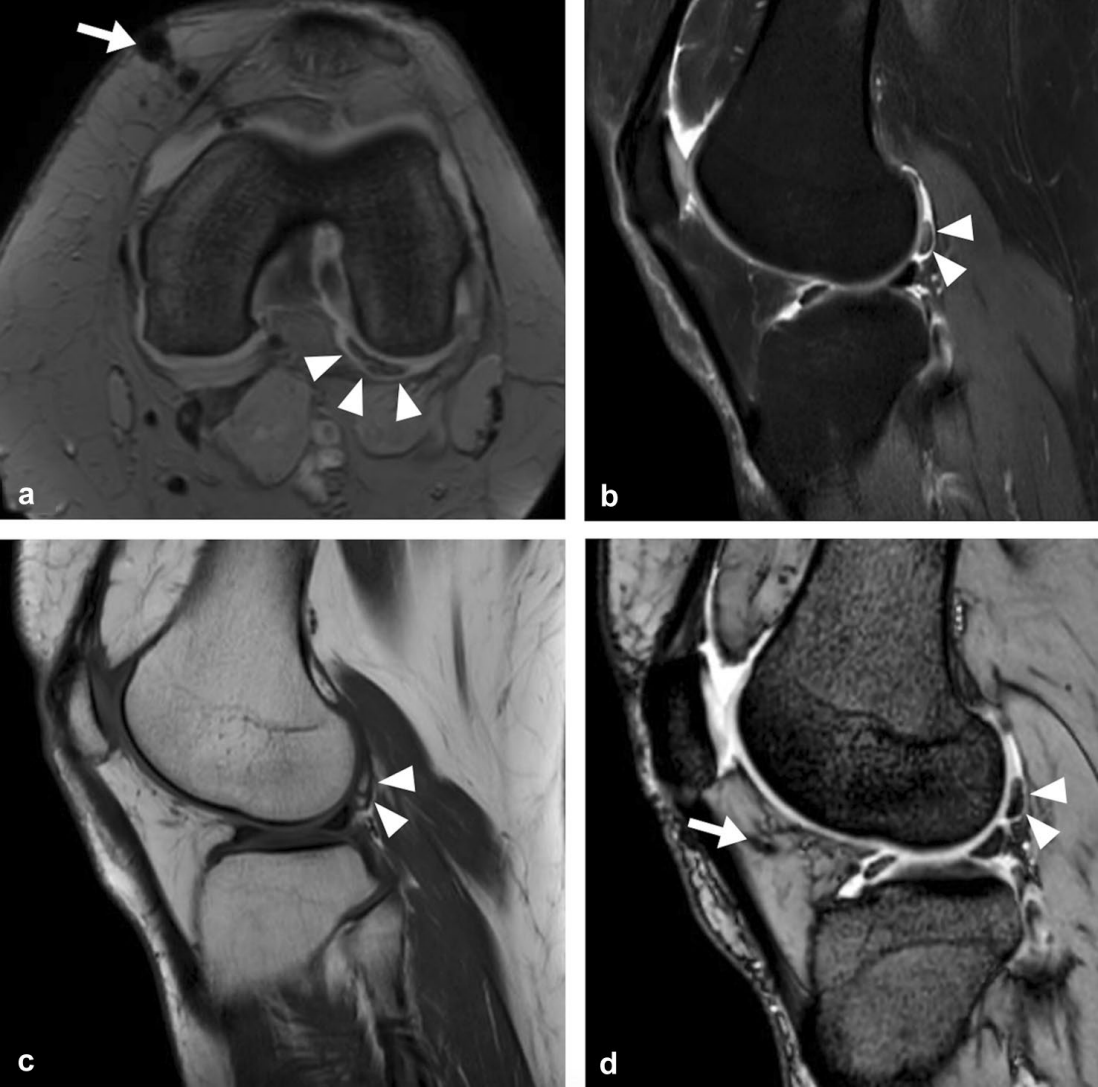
A T2-weighted sm-FFE axial image (Achieva 1.5 T Philips MRI scanner, with dStream technology). A large loose adipocyte intraarticular body in the posterolateral corner circumferentially surrounded by fluid effusion. The intraarticular body is hypointense, well defined (white arrowheads). On the medial anterior side the magnetic susceptibility artifact due to surgical scar (white arrow). B-D sagittal plane (Achieva 1.5 T Philips MRI scanner, with dStream technology): fat sat sequence sm-FFE and mFFE fat sat, T1 weighted TSE confirm adipose content of intraarticular loose bodies, with regular margin and homogenous internal structure that is hypointense with fat saturation and hyperintense in T1 -weighted FSE sequence (white arrowheads). On the anterior side the magnetic susceptibility artifact due to surgical scar (white arrow)
